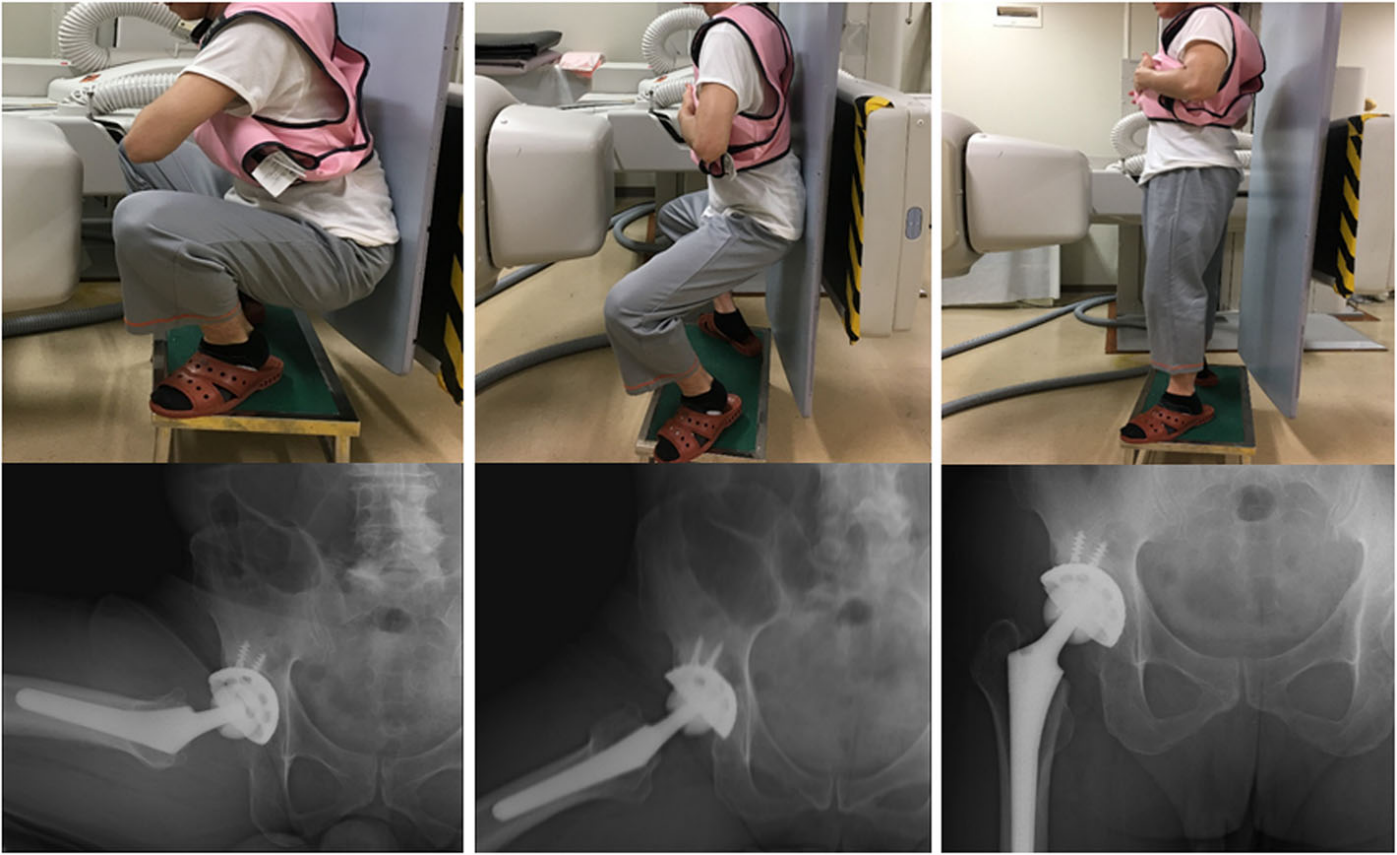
The hip motions during squatting were captured as continuous X-ray images using a flat panel X-ray detector. Patients before and after total hip arthroplasty stood from a squat position with their heel down
